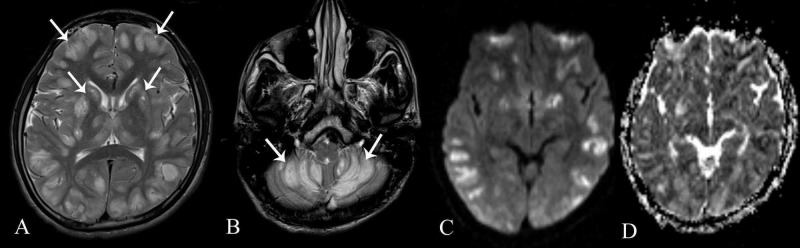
Magnetic Resonance Imaging (A & B) Axial T2-weighted images showing symmetrical abnormal T2 hyperintense signals in cortical grey matter, bilateral caudate nuclei, bilateral putamen, bilateral thalami and bilateral cerebellar hemispheres (white arrows). (C & D) Diffusion-weighted sequences and apparent diffusion coefficient maps demonstrating positive diffusion restriction in these affected areas.
